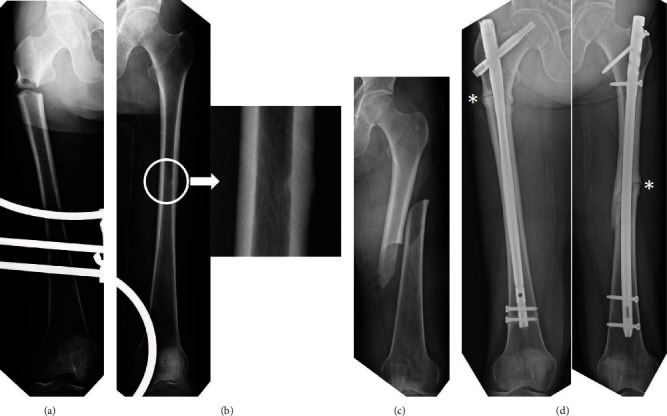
(a) The patient suffered a subtrochanteric AFF without prodromal pain on the right side as a result of a fall. (b) At that time, the left femoral roentgenogram showed periosteal and endosteal thickening of the lateral cortex at the diaphysis (circle; see magnification). (c) Fifteen months later, the patient suffered a diaphyseal AFF on the left side by falling without prodromal pain. (d) A small gap at the lateral cortex of fracture site (asterisk) remained on the right and left sides at 29 and 14 months after the surgery, respectively.
